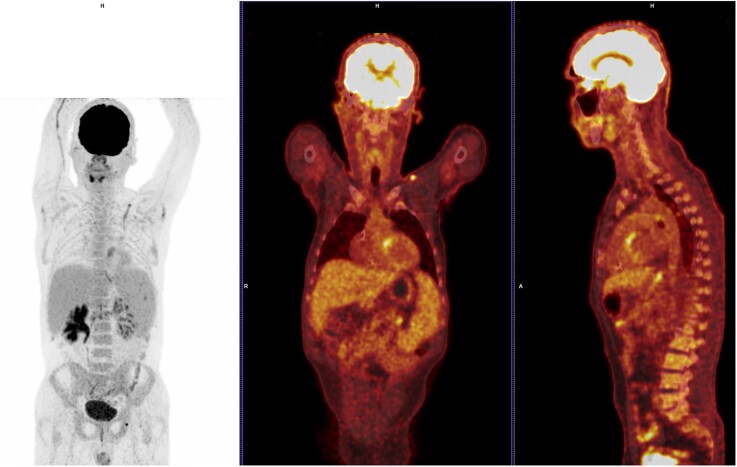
Cardiac implantable electronic device lead infection visualized by fluorine-18 fluorodeoxyglucose positron emission tomography/computed tomography. (*A*) Coronal positron emission tomography images. (*B*) Positron emission tomography fusion images.
